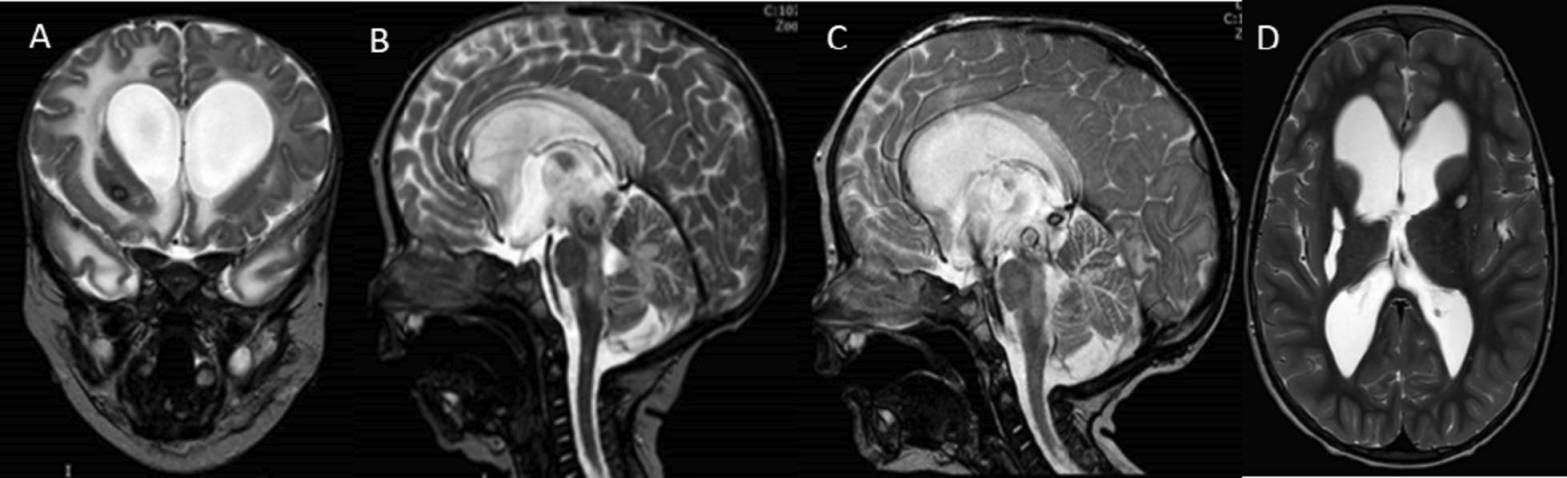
A, B After 48 h of diagnosis: coronal and sagittal T2-weighted images (T2WI) showed a tectal mesencephalic hemorrhage with obstructive hydrocephalus due to aqueductal stenosis associated enlargement of the recesses of the third ventricle as finding of increased intracranial pressure. C After 24 h of surgery, a sagittal T2WI showed the cerebrospinal fluid flow artefact in the third ventricle sign of successful ETV. D After 10 years, a coronal MRI (T2WI) showed residual lacunar sequelae right nucleo-capsual and the regression of vascular lesions
